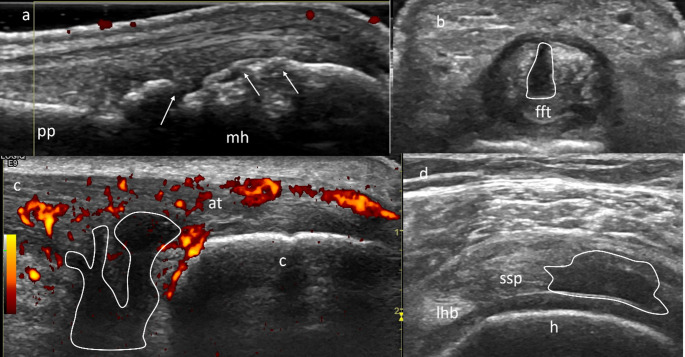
Structural damage at joint and tendon level in patients with PsA. Legend. (a) Dorsal longitudinal image showing severe structural damage at the metacarpal head with multiple bone erosions (arrows) and malalignment in a patient with ongoing pain, without evidence of active synovitis. (b) Transverse ultrasound image of the second finger flexor tendons demonstrates focal hypoechoic changes (outlined by white line) within the superficial finger flexor tendon consistent with a partial-thickness tear. (c) Longitudinal ultrasound image of the Achilles tendon demonstrates preinsertional focal fiber disruption of the deep tendon layers (outlined by white line), consistent with a partial-thickness tear (subsequently confirmed on MRI). Note the presence of surrounding power Doppler signal within the Achilles tendon fibers. (d) Transverse image of the supraspinatus tendon showing a complete loss of normal fibrillar pattern across the tendon thickness (outlined by white line), consistent with a full-thickness tear. Acronyms: at: Achilles tendon; c: calcaneal; fft: finger flexor tendon; mh: metacarpal head; ssp: supraspinatus tendon; lhb: long head of biceps tendon; h: humerus; pp: proximal phalanx
